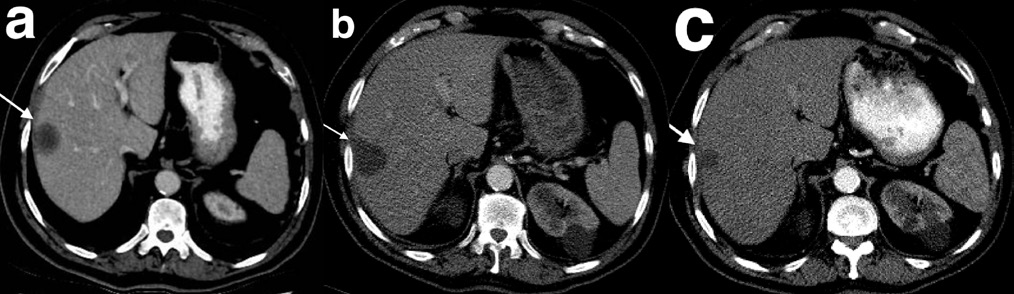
Post-treatment appearances of GIST liver metastases. Portal venous CT performed prior to treatment demonstrates a lesion in segment 5 of the liver which is hypoattenuating with a central area of more marked hypoattenuation (a). Following tyrosine kinase inhibitor therapy, there is a reduction in density from 36 to 9 Hounsfield units and a mild increase in size (b). A further portal venous CT 18 months after the initial CT shows that the lesion remains cystic in attenuation and has decreased in size (c). GIST, gastrointestinal stromal tumour.
